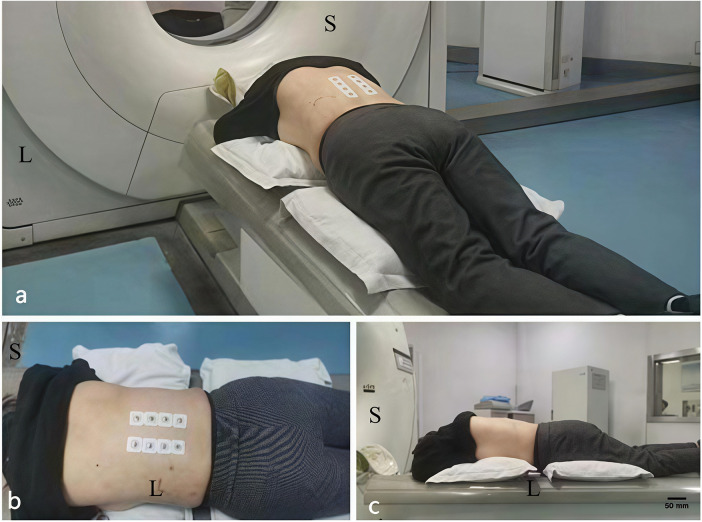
Preoperative CT data acquisition and standardized patient positioning for 3D-printed guide design. (a) Lateral view of the patient in prone position for CT scanning; (b) dorsal view of the patient with fixed positioning electrode pads; (c) lateral view of the standard intraoperative prone position. Orientation: S = Superior, L = Left. Scale bar = 50 mm for the whole figure, calibrated by the standard 33 mm-side-length positioning electrode pads in the images.
